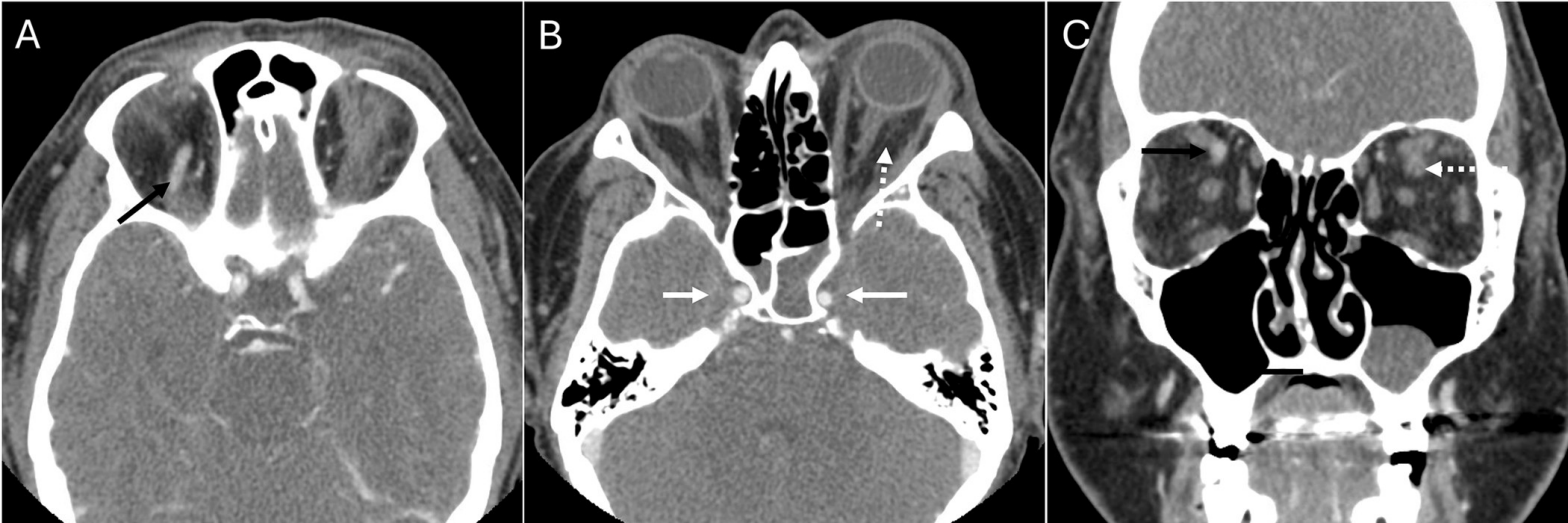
Orbital infection. Axial (A, B) and coronal (C) contrast-enhanced computed tomography scans of a 43 year-old female show left proptosis, post-septal stranding (dashed arrow, B), and a non-enhancing left superior ophthalmic vein (SOV, dashed arrow,C), compared to normally enhanced right SOV (black arrow, A,C). Opacified sphenoid sinuses suggest an infectious source and lack of opacification in the adjacent cavernous sinuses (white arrows, B) raises concern for thrombosis. Both radiologists diagnosed this case as an orbital infection.
